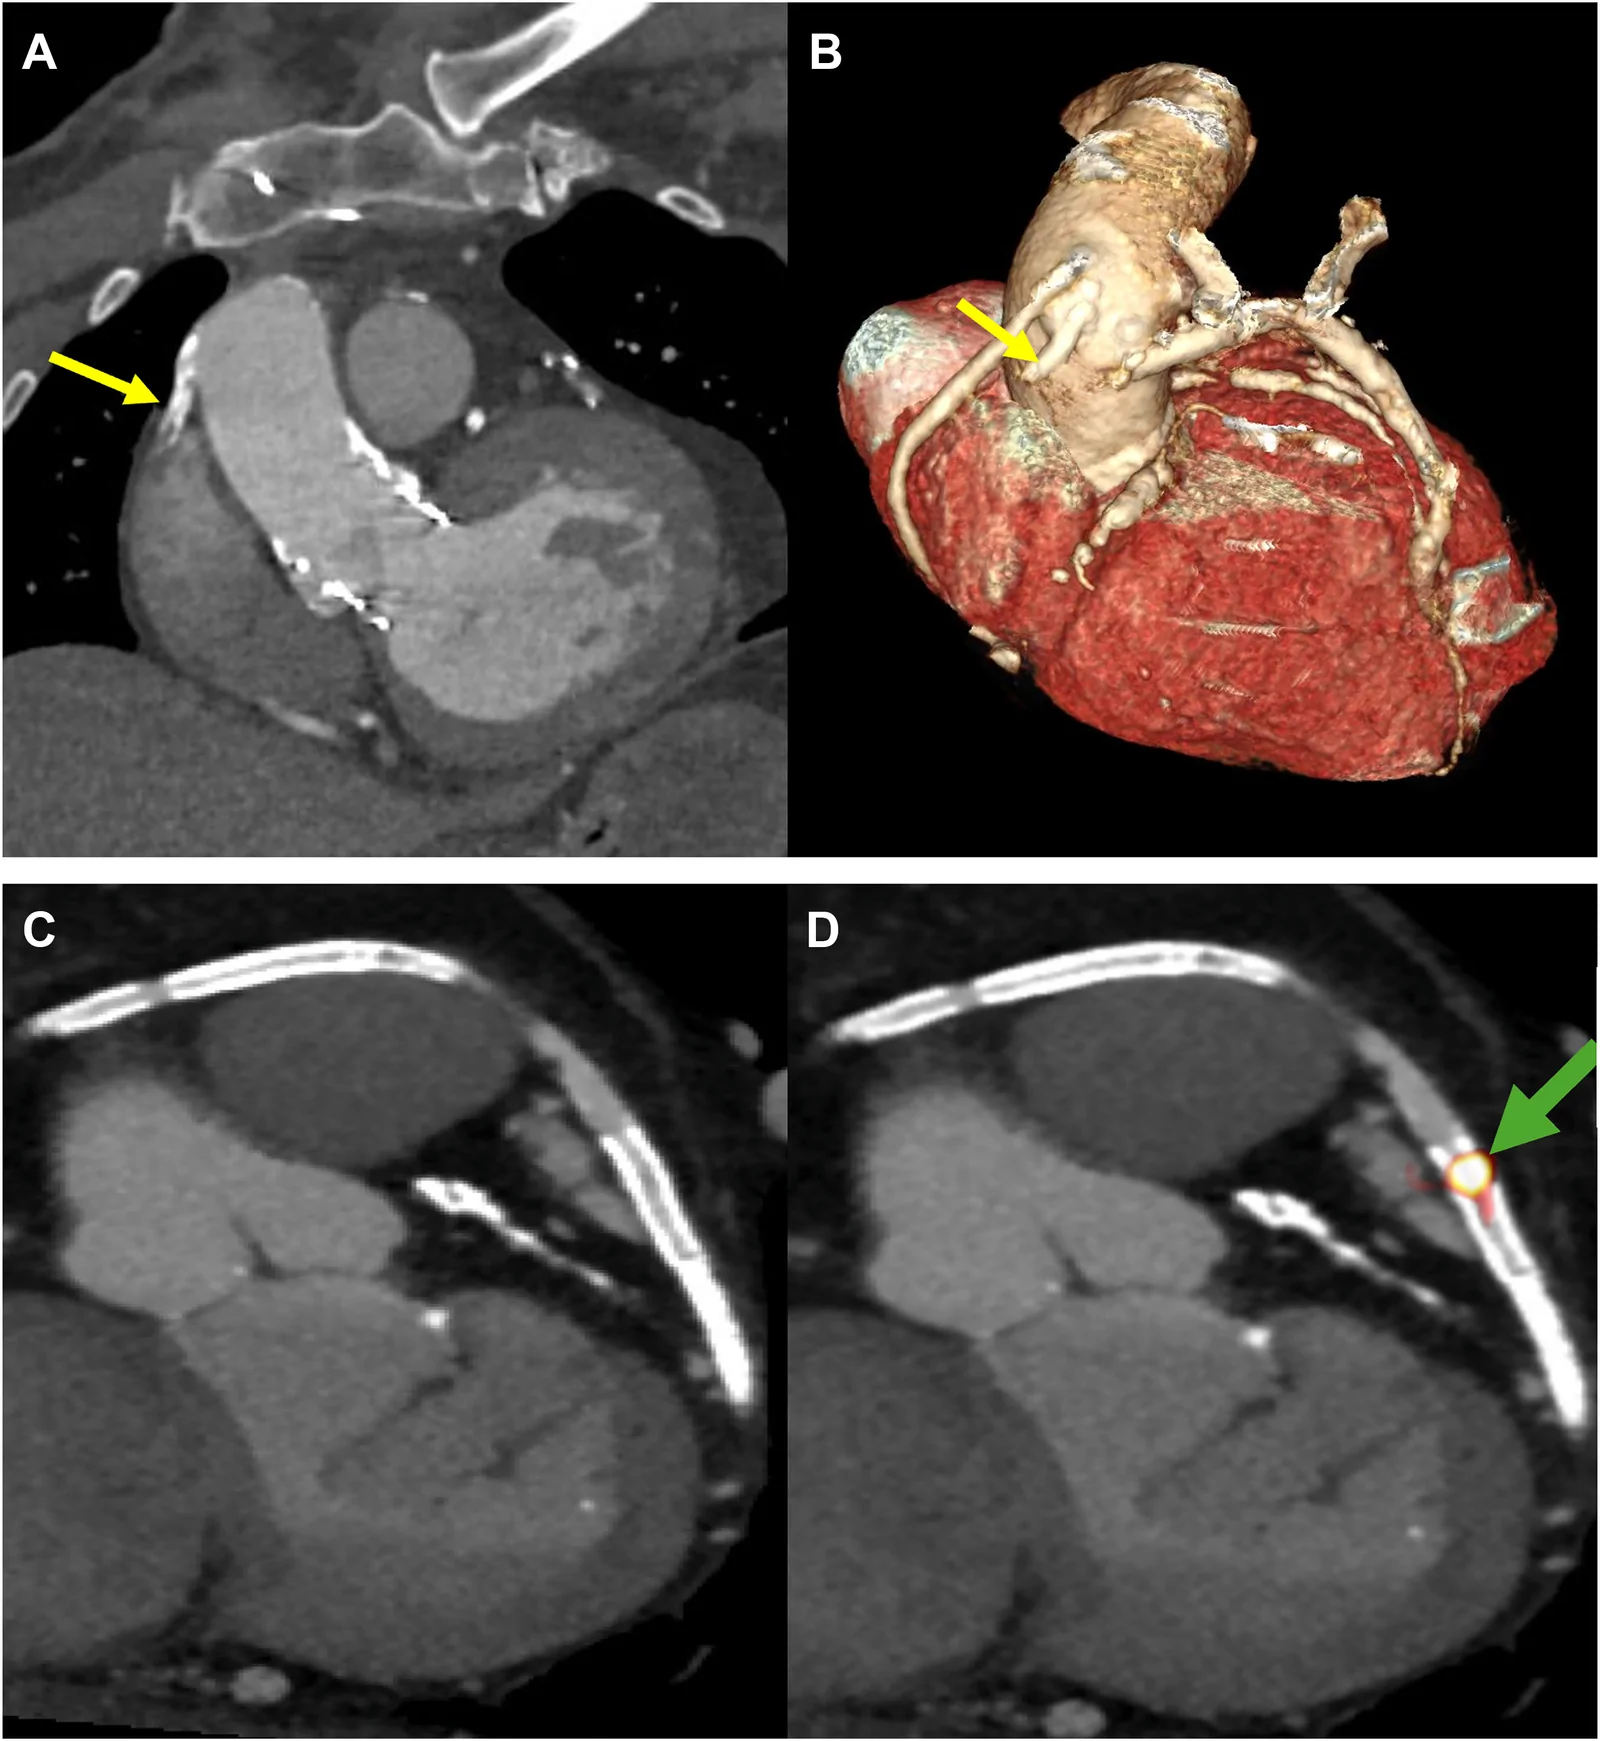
Computed tomography coronary angiography of saphenous vein CABG. (A and B) A patient presenting with stable angina with chronic saphenous vein graft failure after redo coronary artery bypass graft surgery >20 years previously (NCT06800430). Macrocalcification and occlusion of a saphenous vein graft (yellow arrow) going to the right coronary artery on computed tomography coronary angiography. This appears as truncation of the graft on 3D reconstruction, because of the absence of contrast in the distal vessel. (C and D) Adapted from Tzolos et al.^18^ A patient presenting with lateral nonspatial transcriptomics-elevation myocardial infarction and previous coronary artery bypass graft with saphenous vein graft to left circumflex artery. A stented proximal graft (prior procedure) with distal, stented culprit plaque on computed tomography coronary angiography. 18-Fluorine-GP1 uptake is visualized in the culprit lesion of the diseased saphenous vein bypass graft (green arrow)and no uptake is seen in the surrounding vessels.
